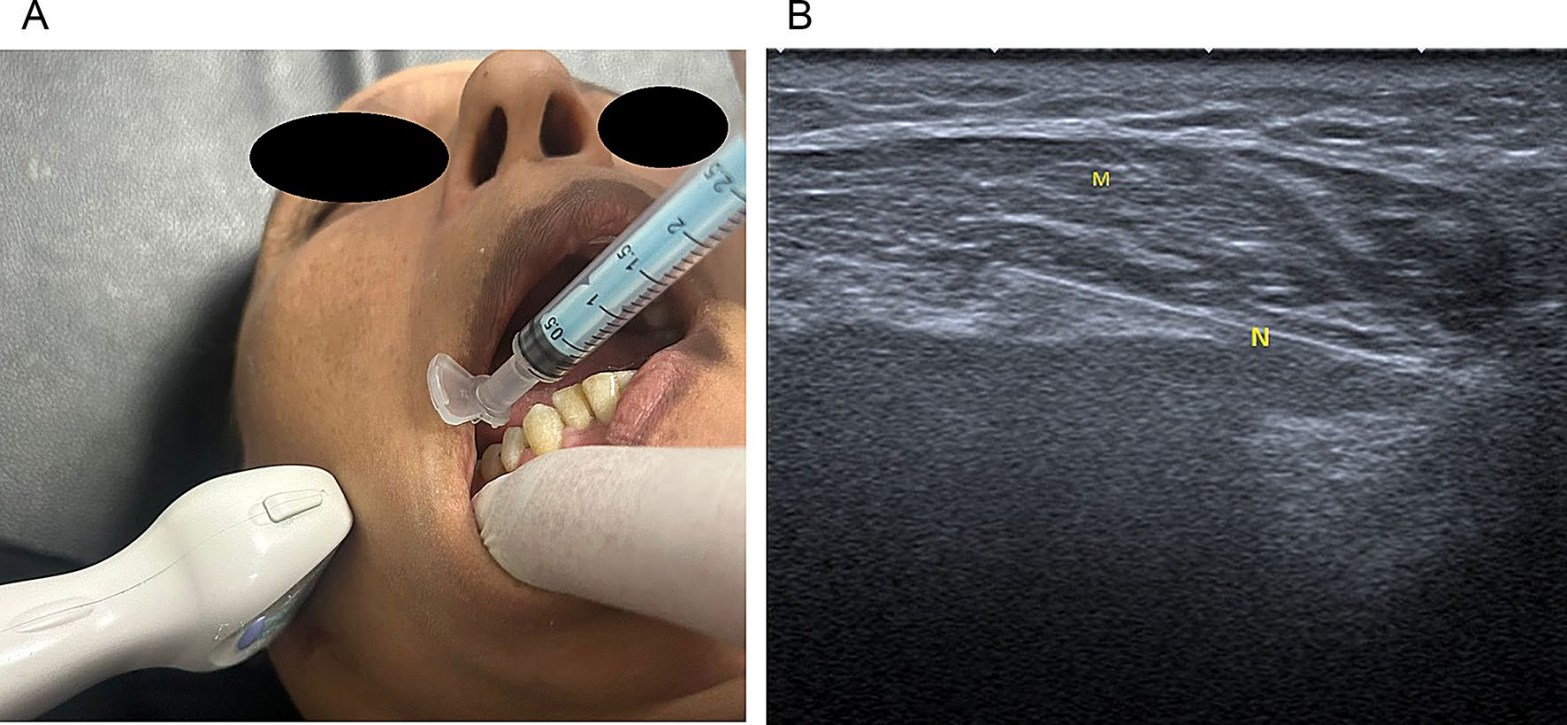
a, b: Showing the intraoral injection with guided ultrasound (N: needle, M: masseter muscle)
